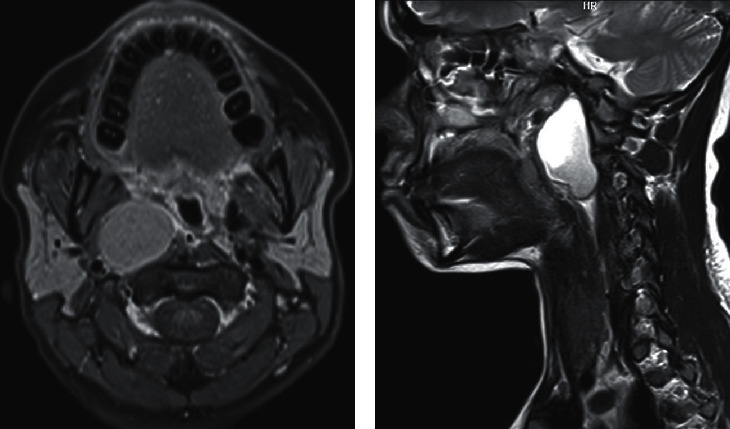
Preoperative magnetic resonance imaging. (a) Axial T1 postcontrast image with fat suppression showing the slightly hyperintense well-defined right parapharyngeal cyst with mild smooth linear wall enhancement and no enhancement of contents, medially indenting nasopharyngeal wall and displacing carotid sheath vessels posterolaterally. (b) Sagittal T2 sequence showing the well-defined cyst extending to skull base and contents heterogeneously hyperintense with fluid-fluid level.
